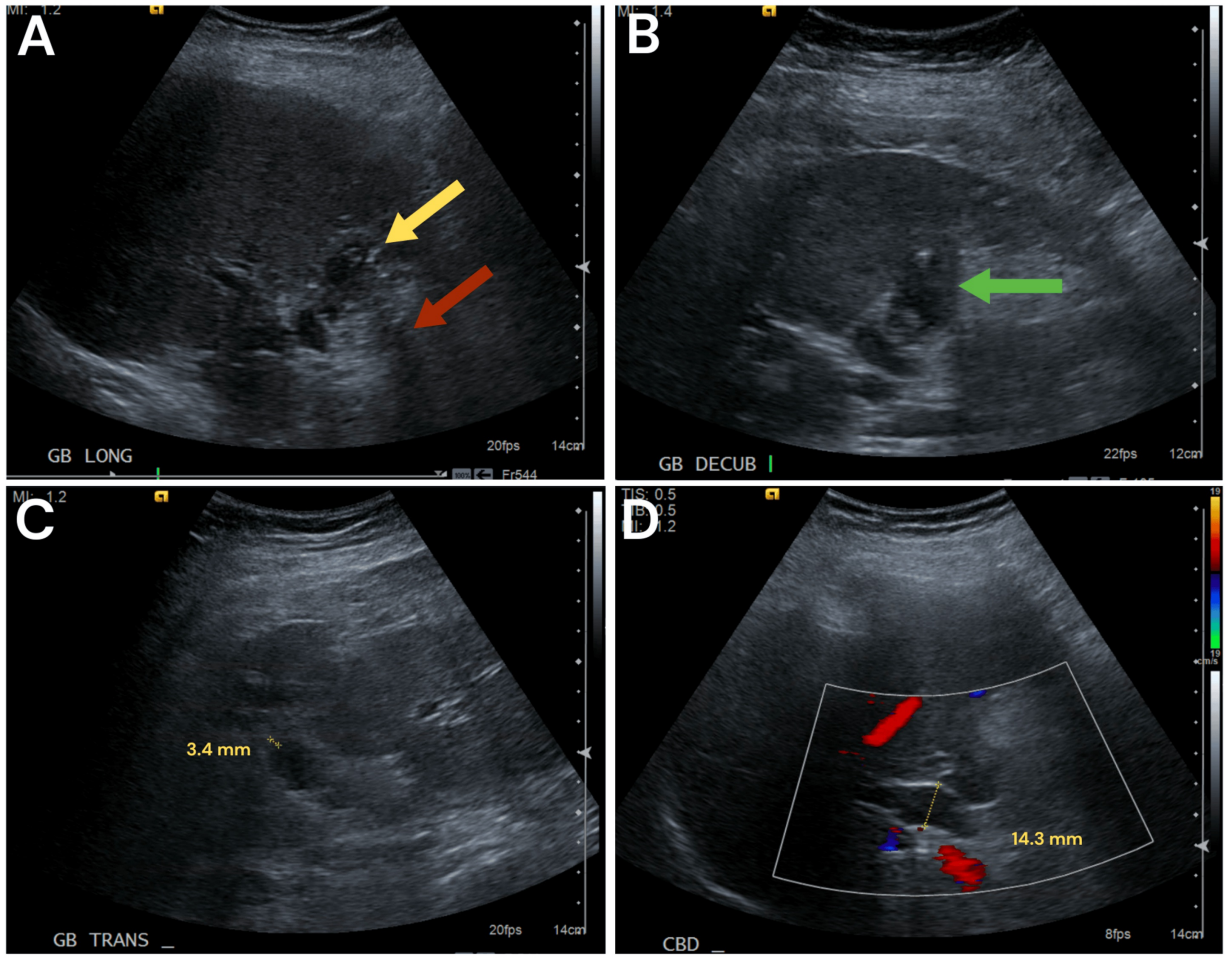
Right upper quadrant ultrasound images (a) Multiple intrinsic echogenic foci (yellow arrow) with acoustic shadowing (red arrow). Ringdown artifact from the margins of the gallbladder. (b) The gallbladder lumen is relatively contracted. No pericholecystic fluid. (c) The gallbladder wall thickness is 3.4 mm, and echogenicity is associated with adenomyomatosis. (d) Common hepatic duct 14.3 mm in anteroposterior (AP) dimension. Intrahepatic bile ducts are not significantly dilated.
